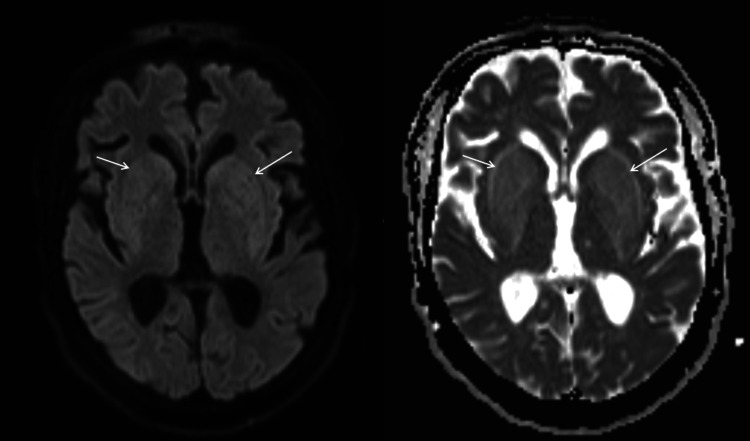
DWI and ADC map images of the brain Diffusion-weighted imaging (DWI) and apparent diffusion coefficient (ADC) map images demonstrate bilateral mild high signal intensity with no diffusion restriction.
